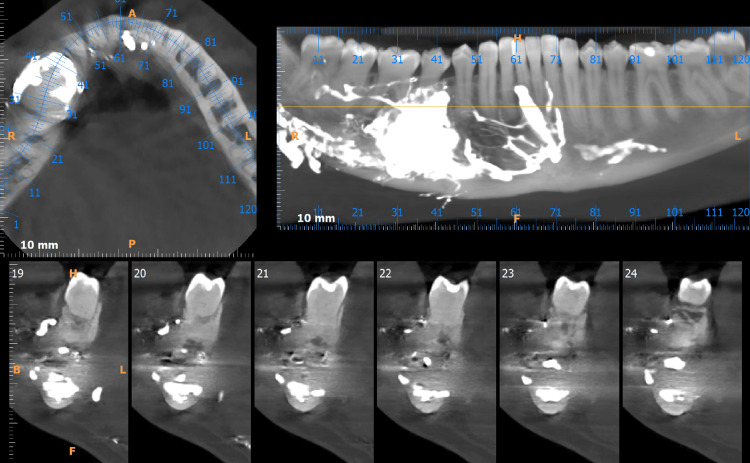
Panoramic radiograph and cone beam computed tomography (CBCT) revealed the embolic agent in the varix.
